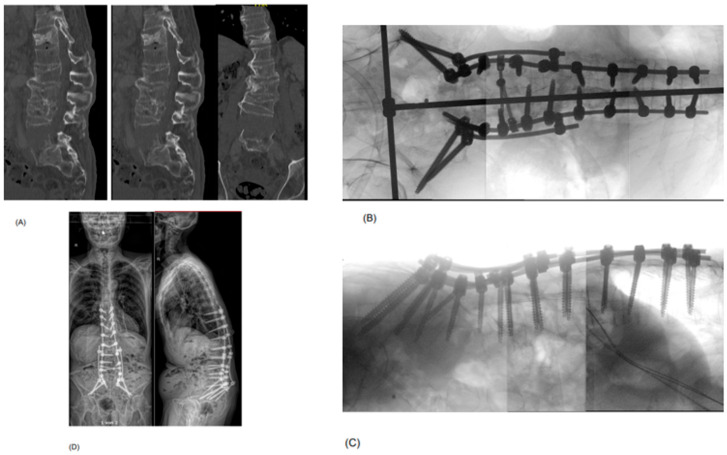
Example of a 73-year-old patient who presented with a B3-type hyperextension injury at the level of the L5 vertebral body in an ankylosed spine, with additional traumatic injuries to the lumbar spine (old L4 incomplete burst fracture (A3) with acutely ruptured L3/4 disc and L4 pedicle fracture, acute Th12 complete burst fracture (A4)) and abdominal organs (e.g., suspected common iliac vein injury) after a motorcycle accident. He was neurologically intact. As the injury was at the level of the L5 vertebral body, an LLIF to fill up the gapping hyperextension injury was not considered possible. Additionally, the retroperitoneal hemorrhage by a venous injury—treated conservatively—hindered an anterior or lateral approach to provide anterior column support by a spacer. (A) shows the preoperative sagittal and axial CT scan post-trauma, demonstrating the extension injury of L5 (chance fracture with craniocaudal dislocation), complete burst fracture of Th12, rupture of the disc space L3/4 and fracture of the pedicle L4 on the right side. (B,C) demonstrate the intraoperative imaging after stabilizing the fractures by a T10-pelvis fusion construct with a four-rod system (S2Ai and iliac screws). (D) shows the standing whole-spine EOS X-ray in ap and lateral view at 3 months postoperative. Note how the gapping defect of L5 has closed to some extent over time, without the anterior column support, resulting in loss of lordosis (38.5°) compared to the intraoperative view.
